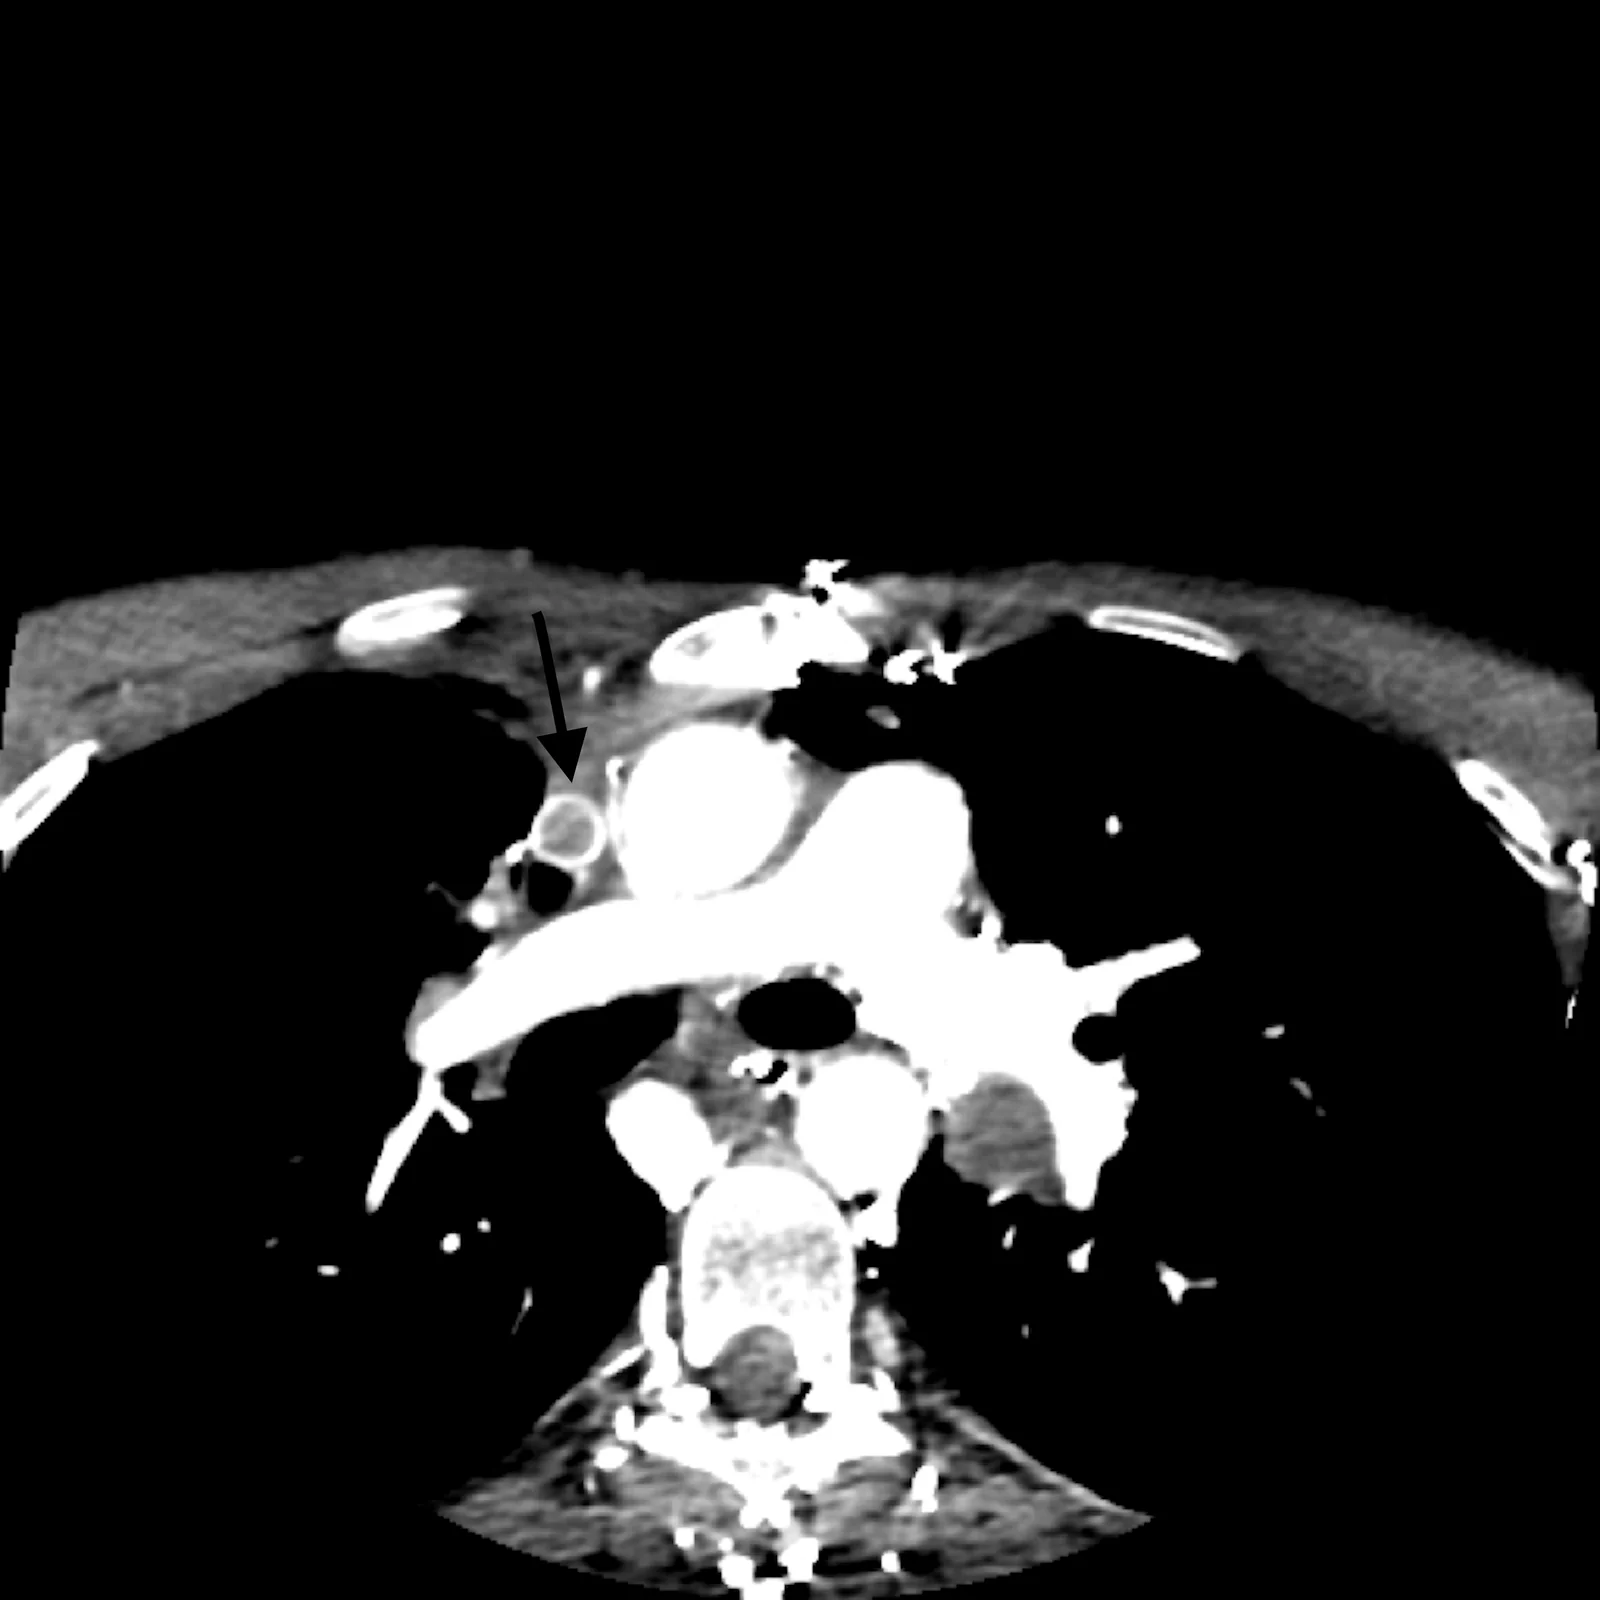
Postoperative contrast-enhanced chest CT axial view showing a patent SVC/PTFE graft SVC: superior vena cava; PTFE: polytetrafluoroethylene
